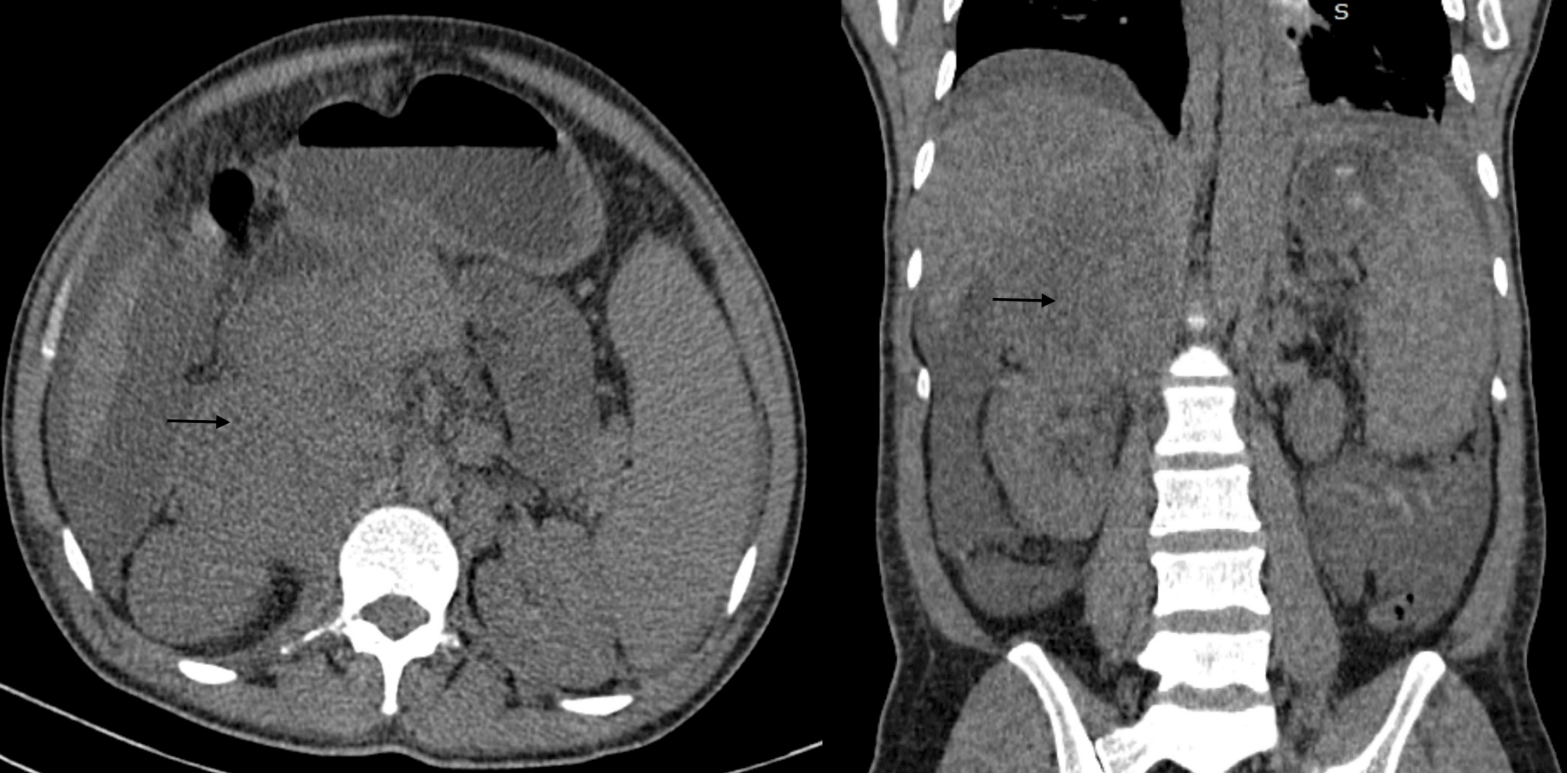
Pre-contrast CT revealing a large right adrenal mass (black arrows)
